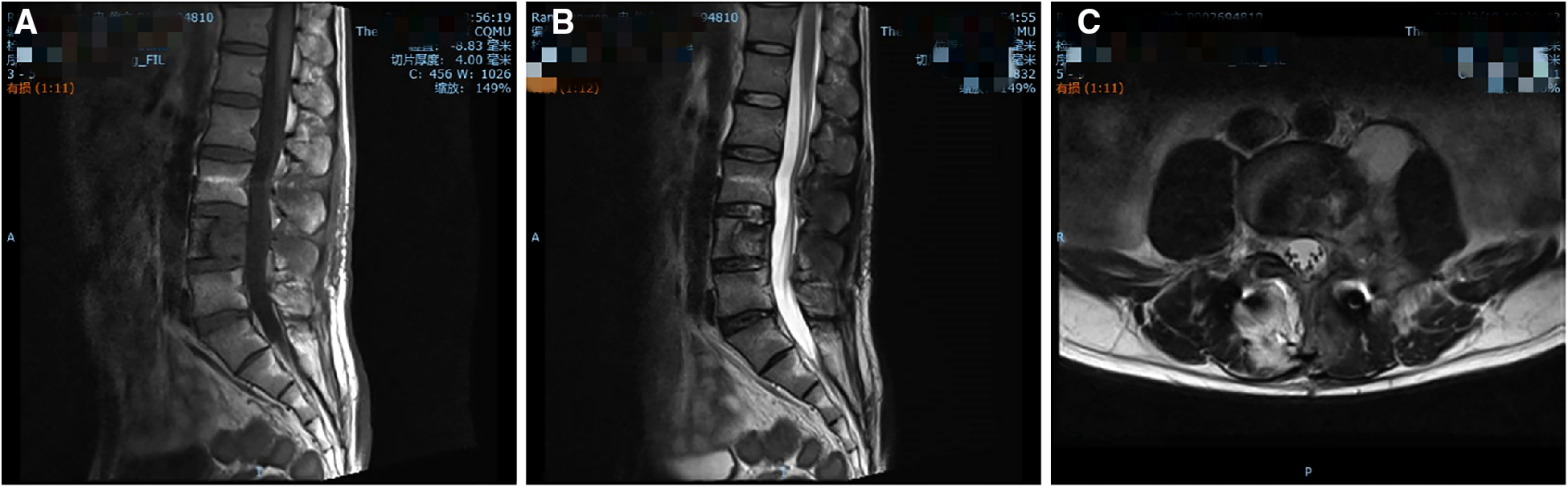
Twelve-month follow-up MRI shows a significant reduction of the lesion. (A) T1-weighted, (B) T2-weighted, and (C) axial images.
